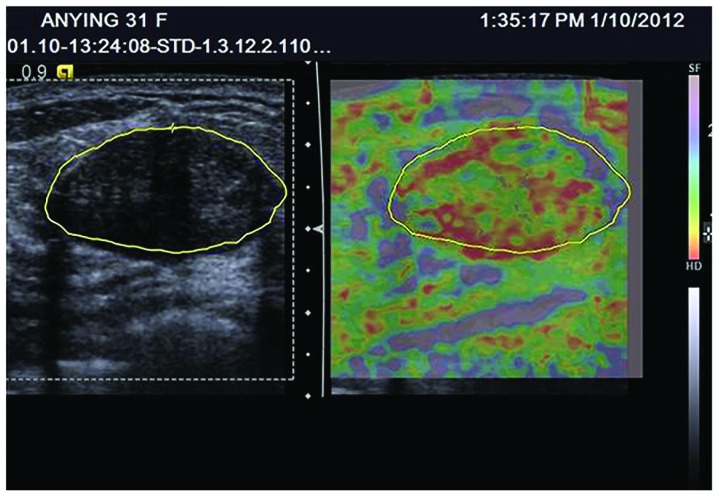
Surgical pathology in a 31-year-old woman with a palpable mass in the inner upper quadrant of her left breast proved an adenofibroma. Left image: Two dimensional sonogram. Right image: Elastography map, 3 points.
